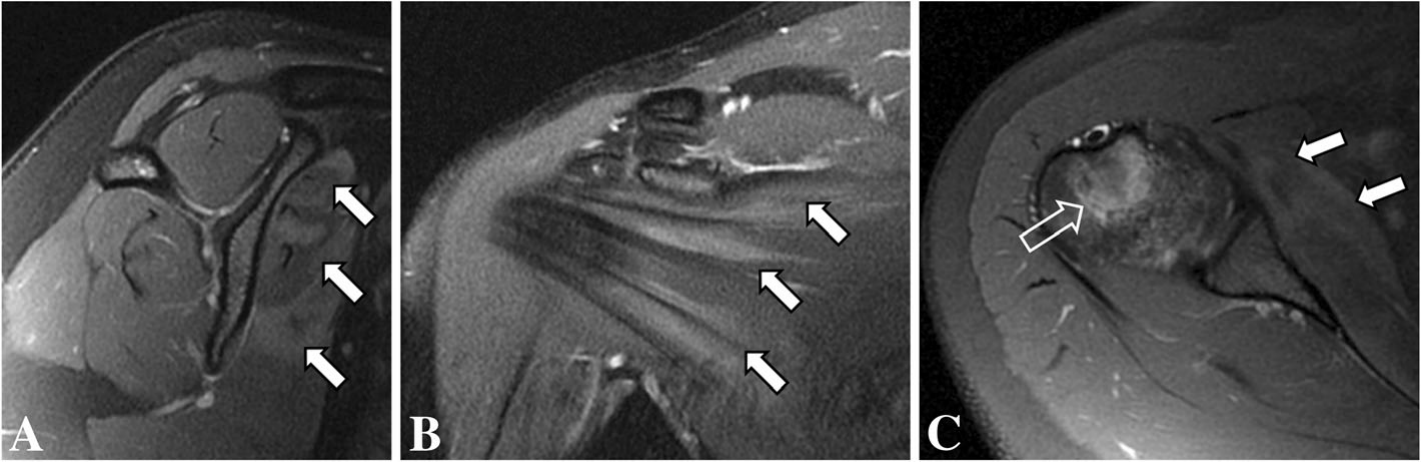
Sagittal (a), coronal (b) and axial (c) T2-weighted fat-suppressed MR images of the right shoulder showing intense bone marrow edema of the humerus (open arrow), with edema of the subscapularis muscle (arrows)
